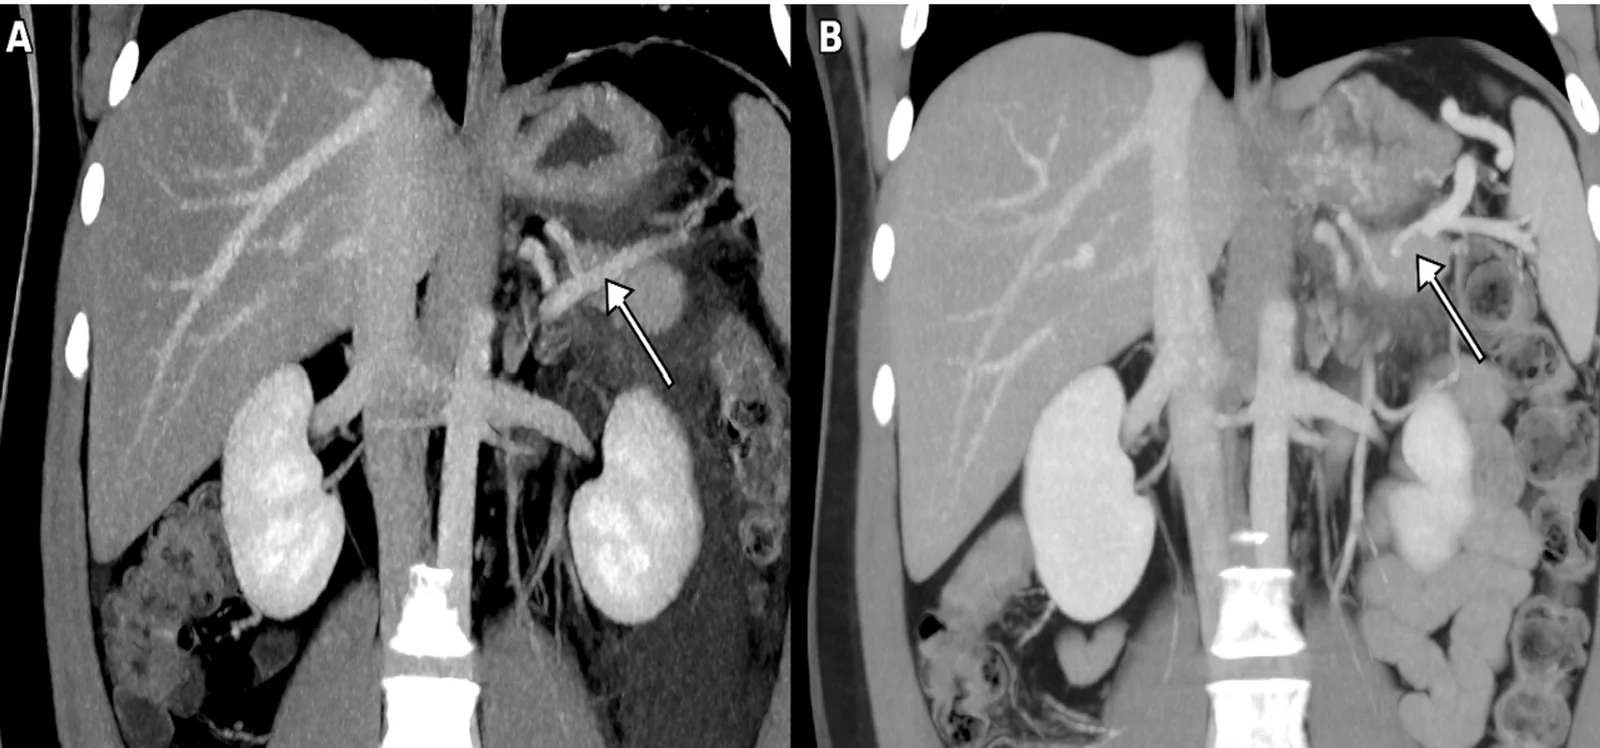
Coronal contrast-enhanced computed tomography, matched magnification. (A) Baseline: Patent, uniformly opacified splenic vein. (B) Day 3: Abrupt cutoff of the splenic vein with a non-enhancing intraluminal filling defect and hilar collateral vessels (arrow), consistent with splenic vein thrombosis.
